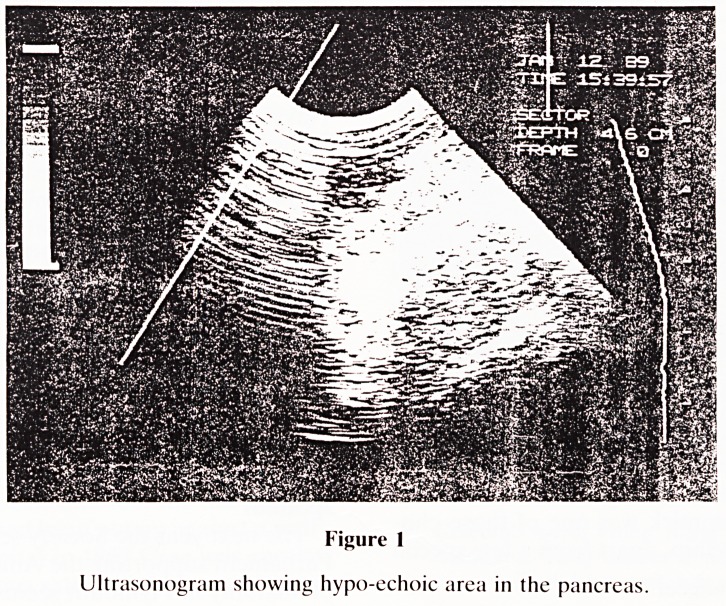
# Intra-Operative Ultrasound in the Surgery of Insulinomas
